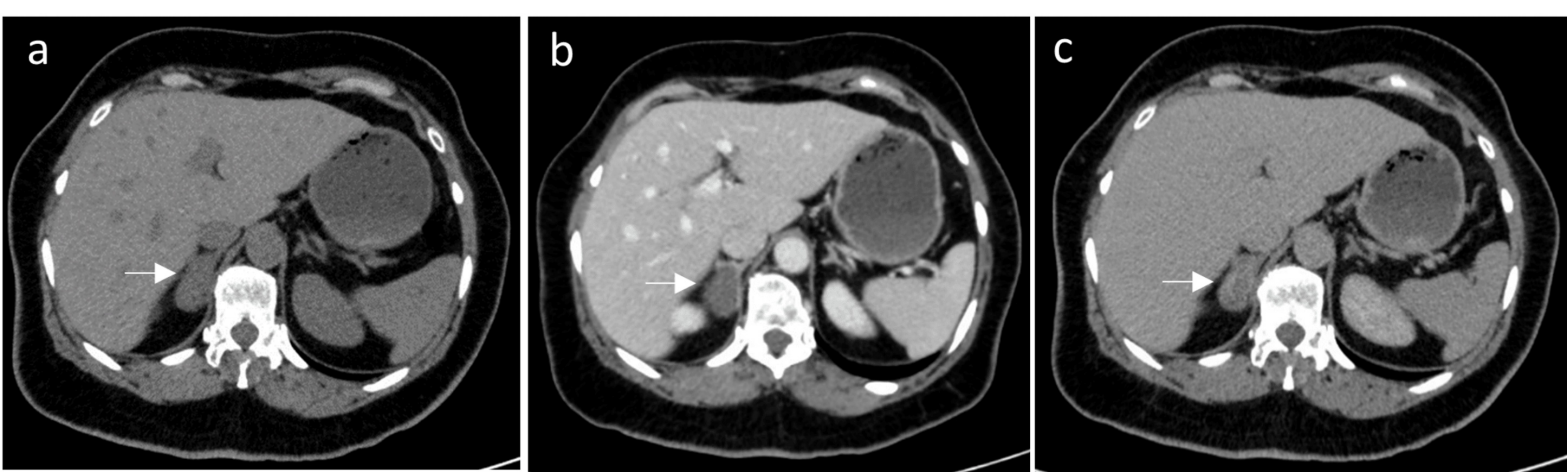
Computed tomography scan of the abdomen with adrenal protocol. Computed tomography scan of the abdomen with adrenal protocol showing a right adrenal mass (Arrow) measuring 3.2 x 1.8 cm in the a) pre-contrast phase, b) portovenous phase, and c) delayed phase.
